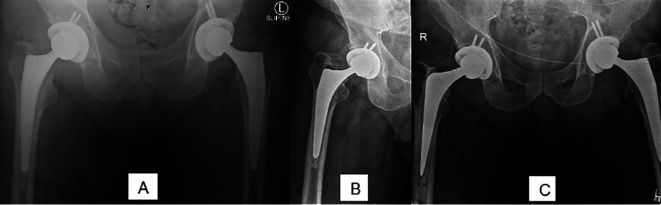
Right femoral stem subsidence in patient with myeloproliferative disorder. (A) Initial plain radiograph. (B) Subsidence at 1.5 year. (C) Plain radiograph post revision arthroplasty with cemented CPCS.
